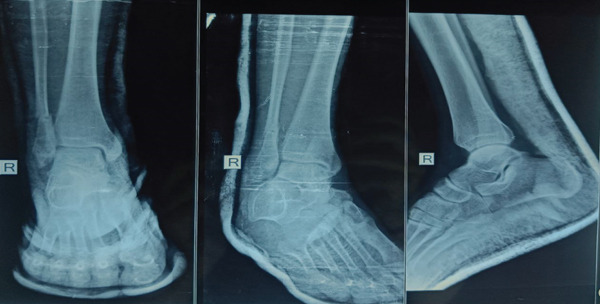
Anteroposterior, mortise, and lateral plain radiographs showing the patient′s right limb immobilized in a below‐knee slab.
